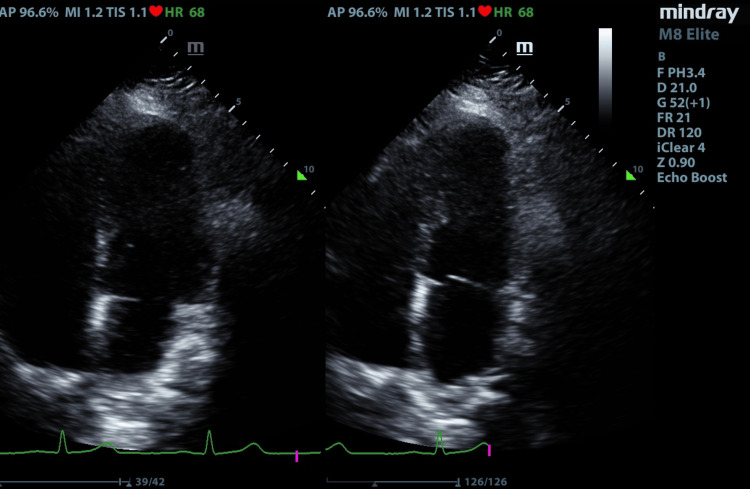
Two-dimensional transthoracic echocardiogram
